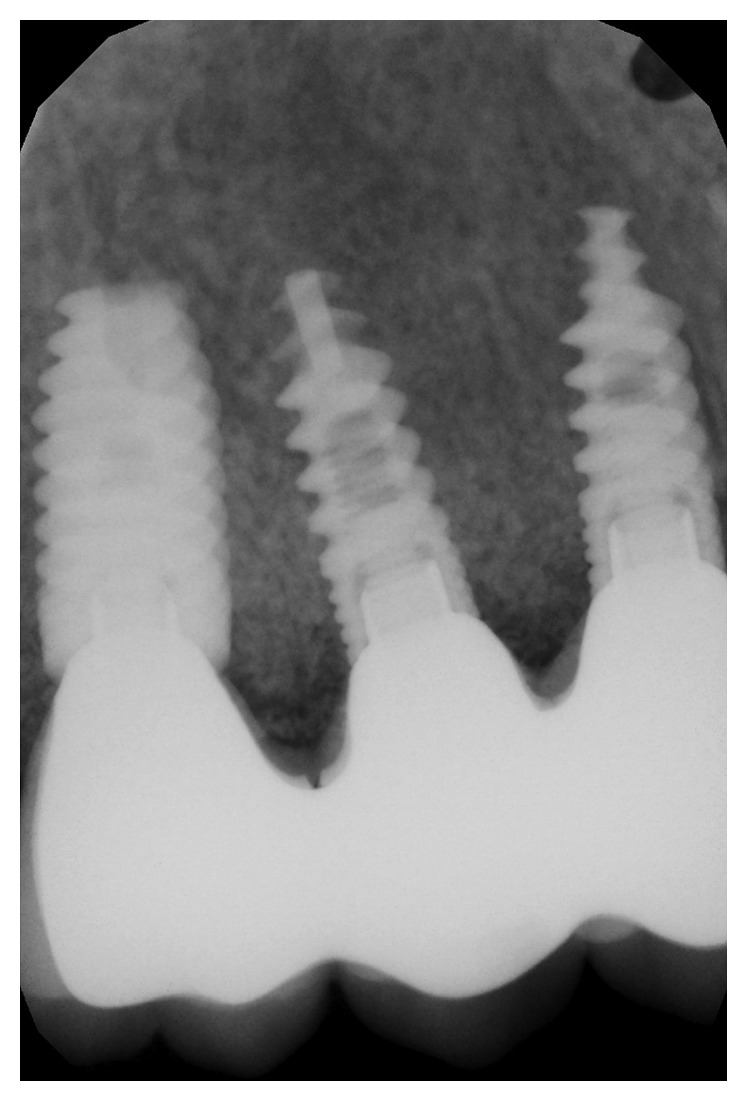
Prosthetic delivery radiograph.
